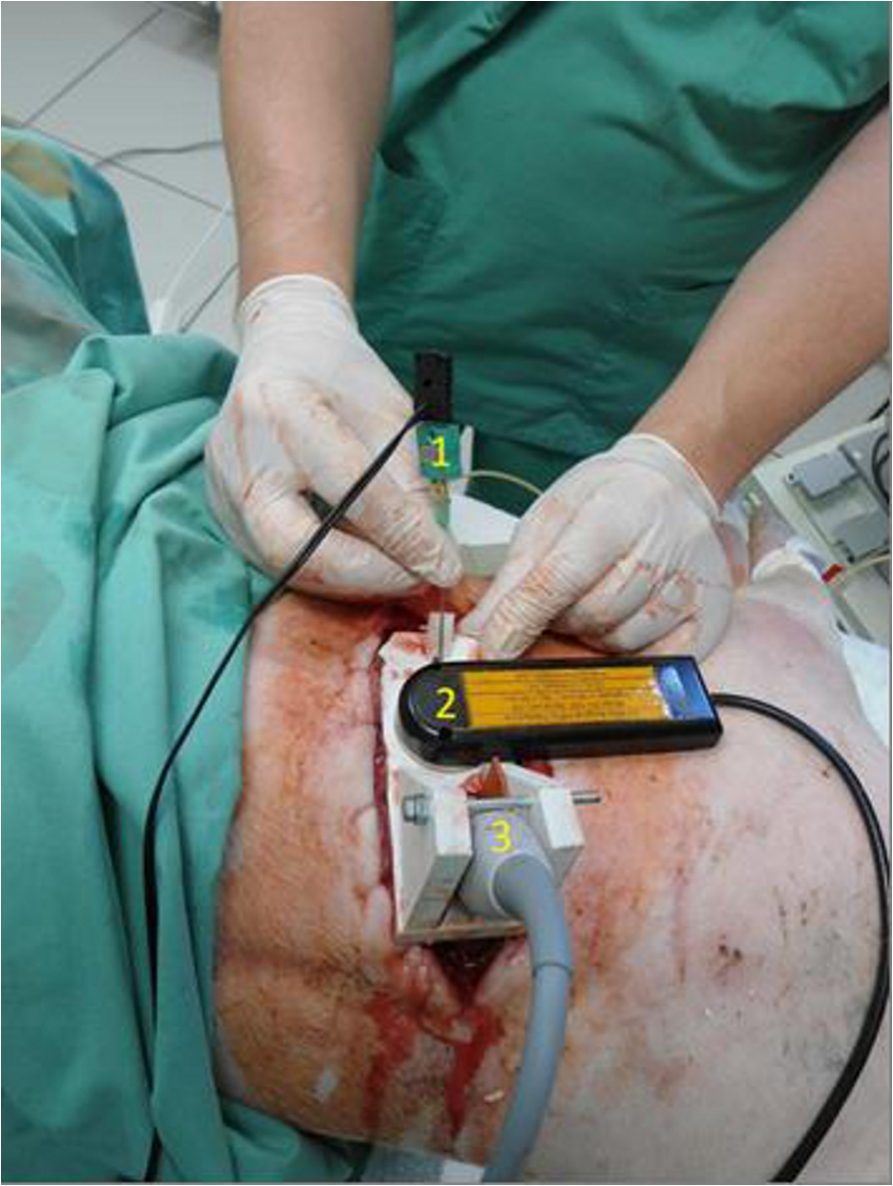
In vivo *high-intensity focused ultrasound application after rib resection. Thermocouple (1), high-intensity focused ultrasound transducer (2), and sector-array probe (C11e, 8 to 5 MHz, Micromaxx, Sonosite Inc., Bothell, WA, USA) (3).
